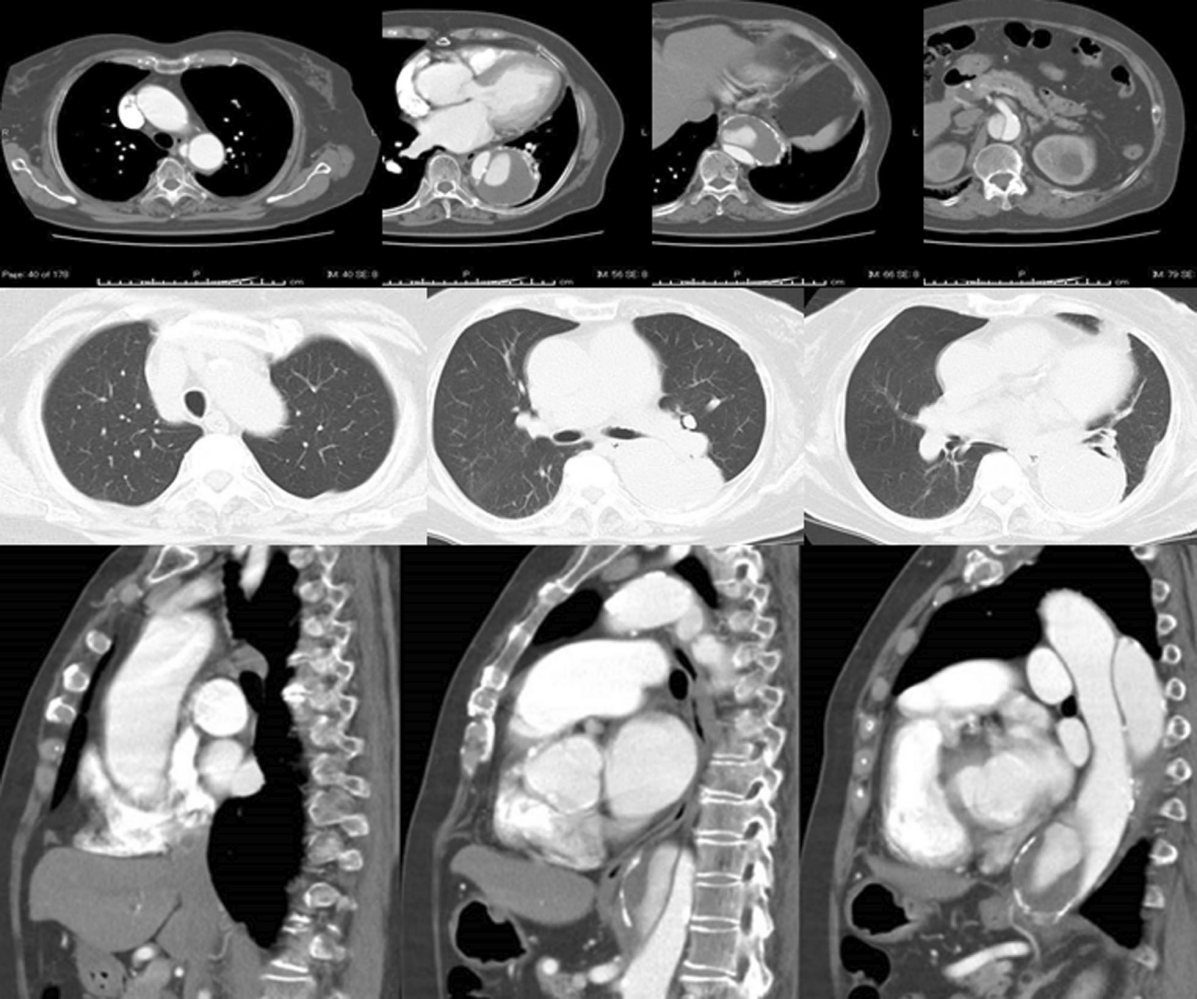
Upper: Enhanced computed tomography imaging. A preoperative CT scan showed chronic type B aortic dissection with a partially thrombosed false lumen. Calcification of the aortic wall on the distal thoracic aorta was observed. The maximum diameter of the aorta was 6.5 cm. Middle: The lung window setting showed no significant findings. Lower: Sagittal view of the enhanced CT imaging
